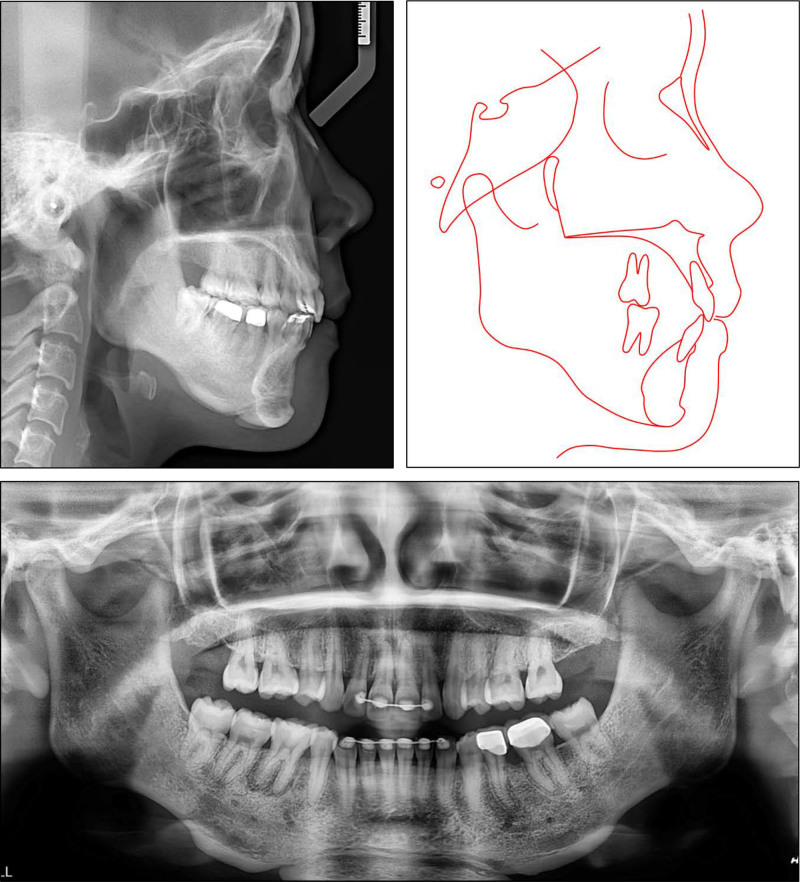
Posttreatment radiographs and tracing.
